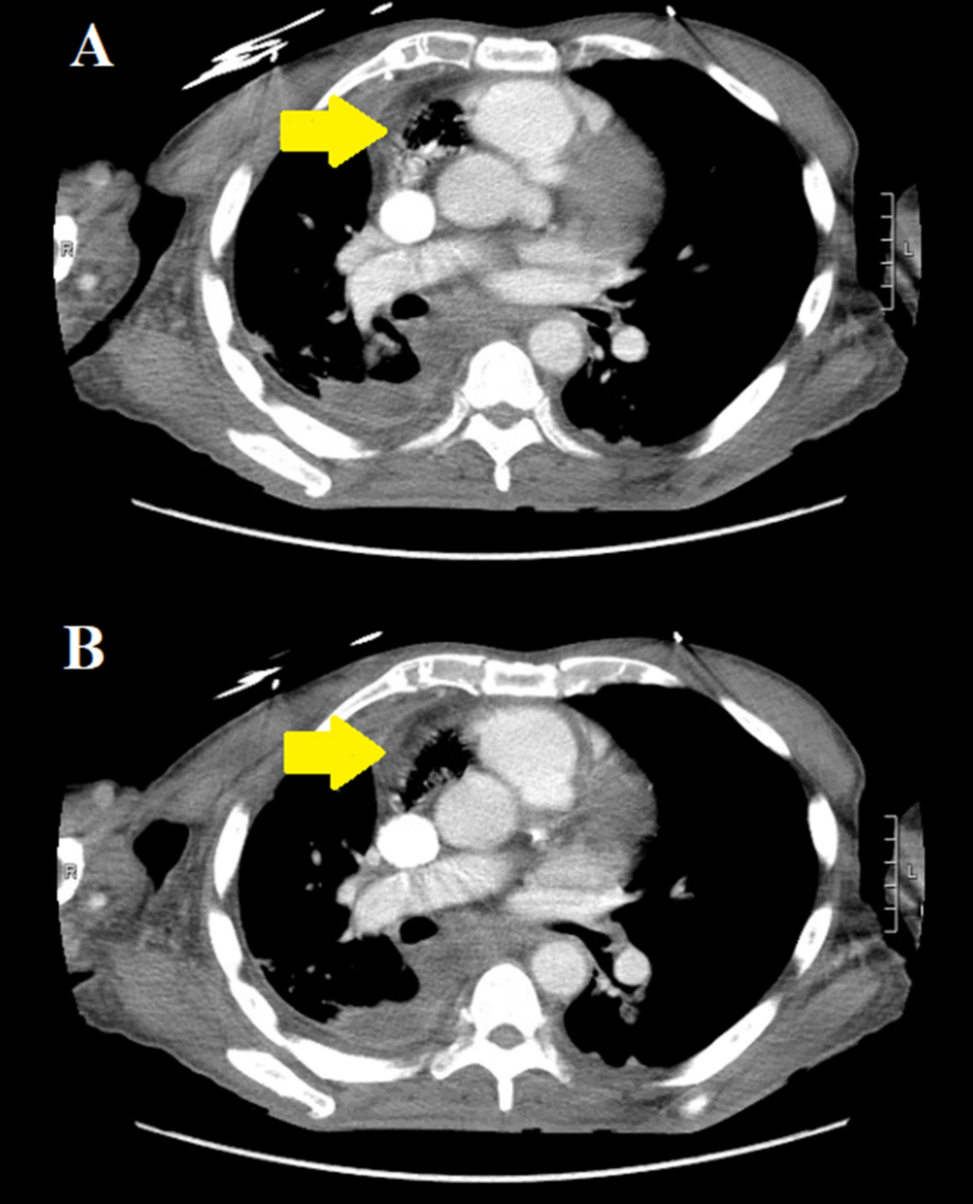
Axial chest CT with intravenous contrast demonstrating a large amount of gas in the nondependent areas of the right atrium (yellow arrows in panes A and B).
